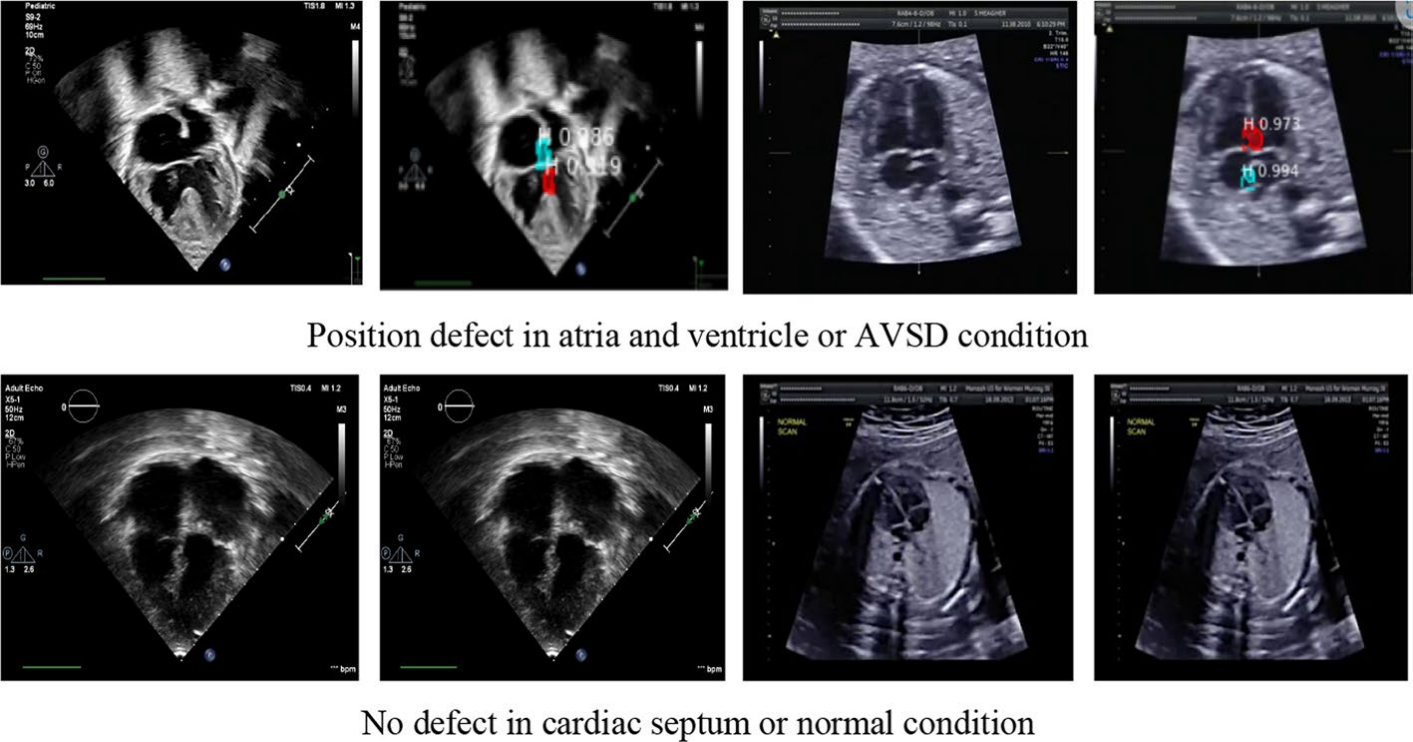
Cardiac septal defect classification based on prenatal and postnatal echocardiograms. The red area in the wall chamber is the defect with confidence value from the instance segmentation process
